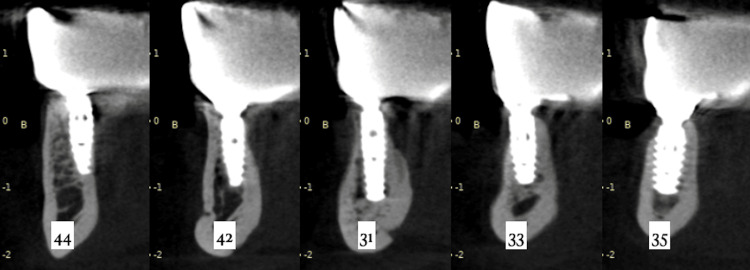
CBCT of the implants at the 24-month follow-up CBCT: Cone beam computed tomography
